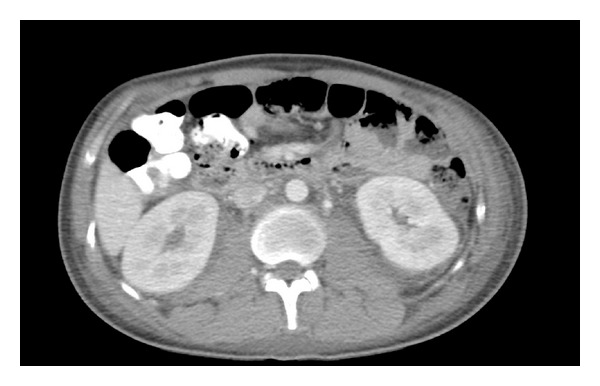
CT abdomen demonstrating extensive retroperitoneal fat stranding. These changes were later determined on autopsy to represent abdominal involvement of ALCL, which was present in mesenteric and retroperitoneal lymph nodes.
